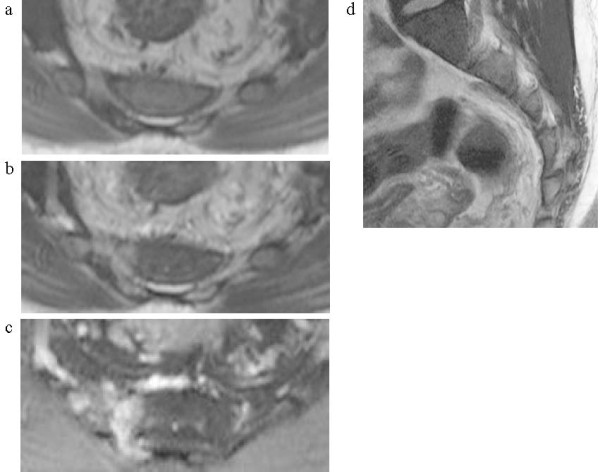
Magnetic resonance imaging scan of the sacrum. On the magnetic resonance imaging scan, the tumor was depicted as a low-intensity lesion on T1-weighted images (a) and high-intensity lesion on both T2-weighted images (b) and T2-weighted selective partial inversion recovery images (c, d).
